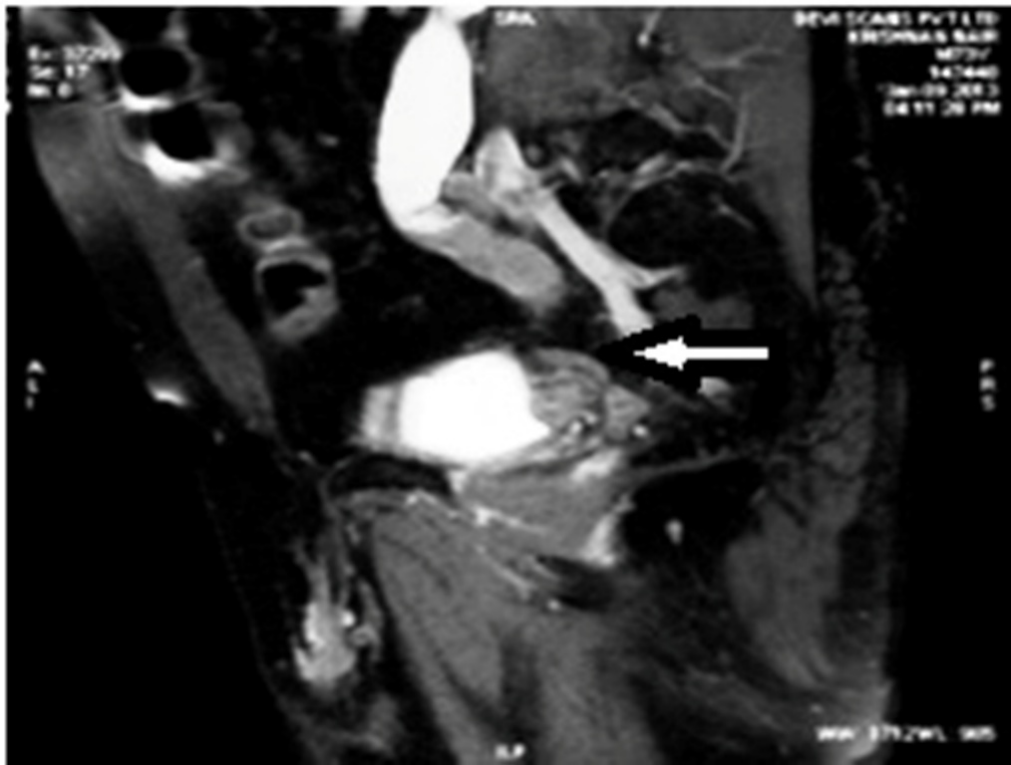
Sagittal T1 weighted post-contrast magnetic resonance image showing an irregular filling defect within the distal ureter with proximal dilatation. The distal ureter appears to be completely occluded by the lesion.
